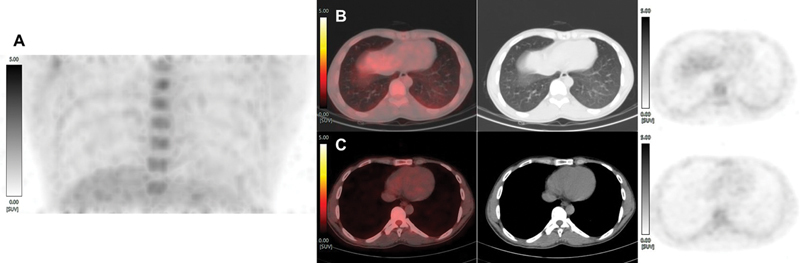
Maximum intensity projection of a repeat F-18 2-fluoro 2-deoxyglucose positron emission tomography with noncontrast computed tomography ([
